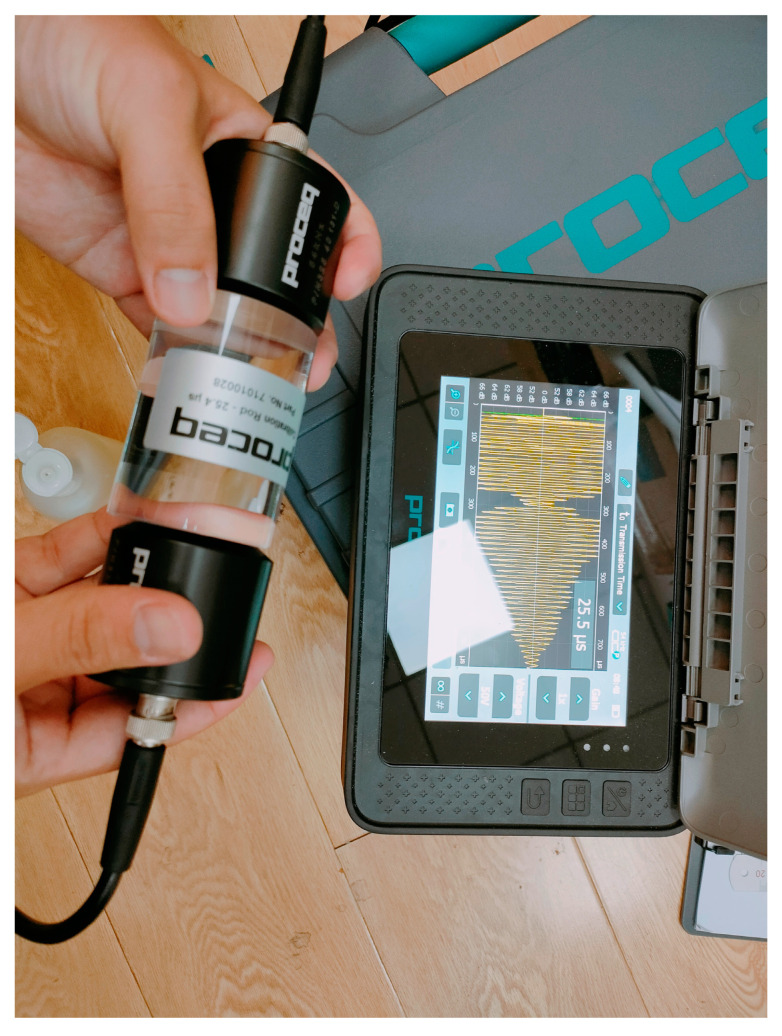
Ultrasonic non-destructive testing equipment.
